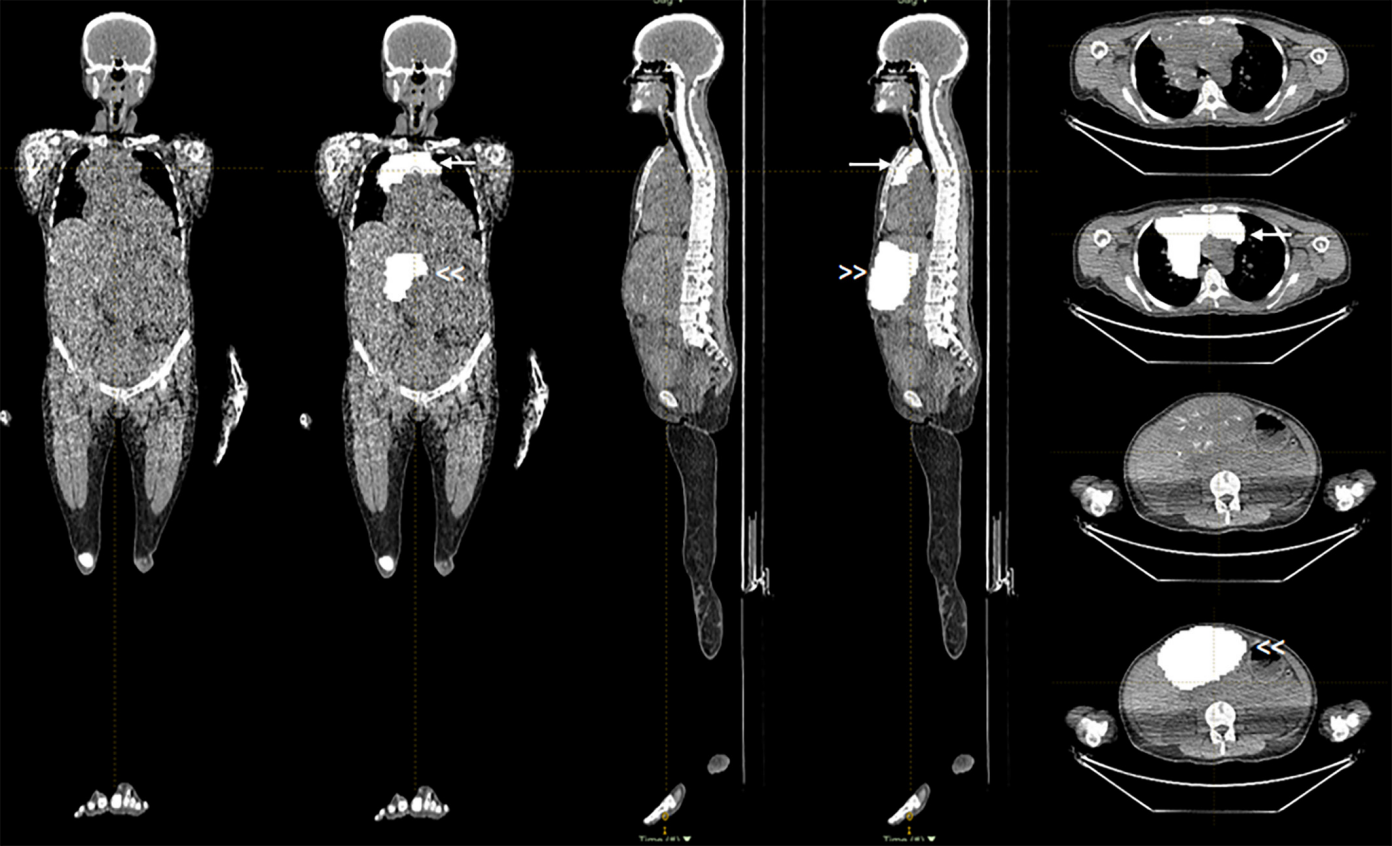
Coronal, sagittal, and axial images of the CT component of the ^18^F-NaF PET/CT study showing the extraosseous lesions without and with the VOIs at the level of the mediastinum (arrow) and liver (double arrowhead).
